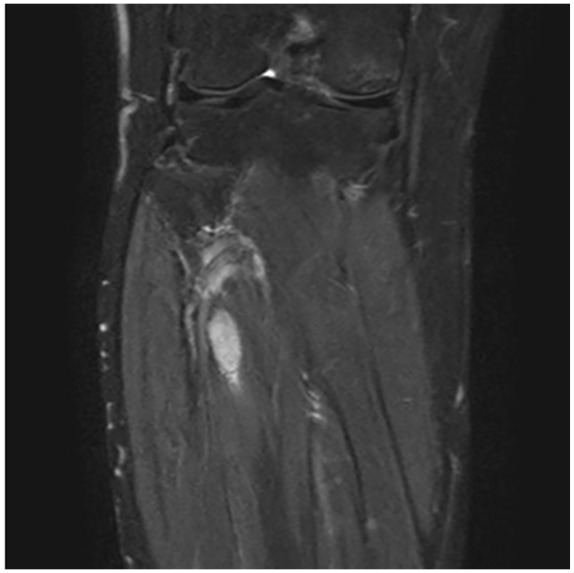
Magnetic resonance imaging of solid intramuscular tumor corresponding to
